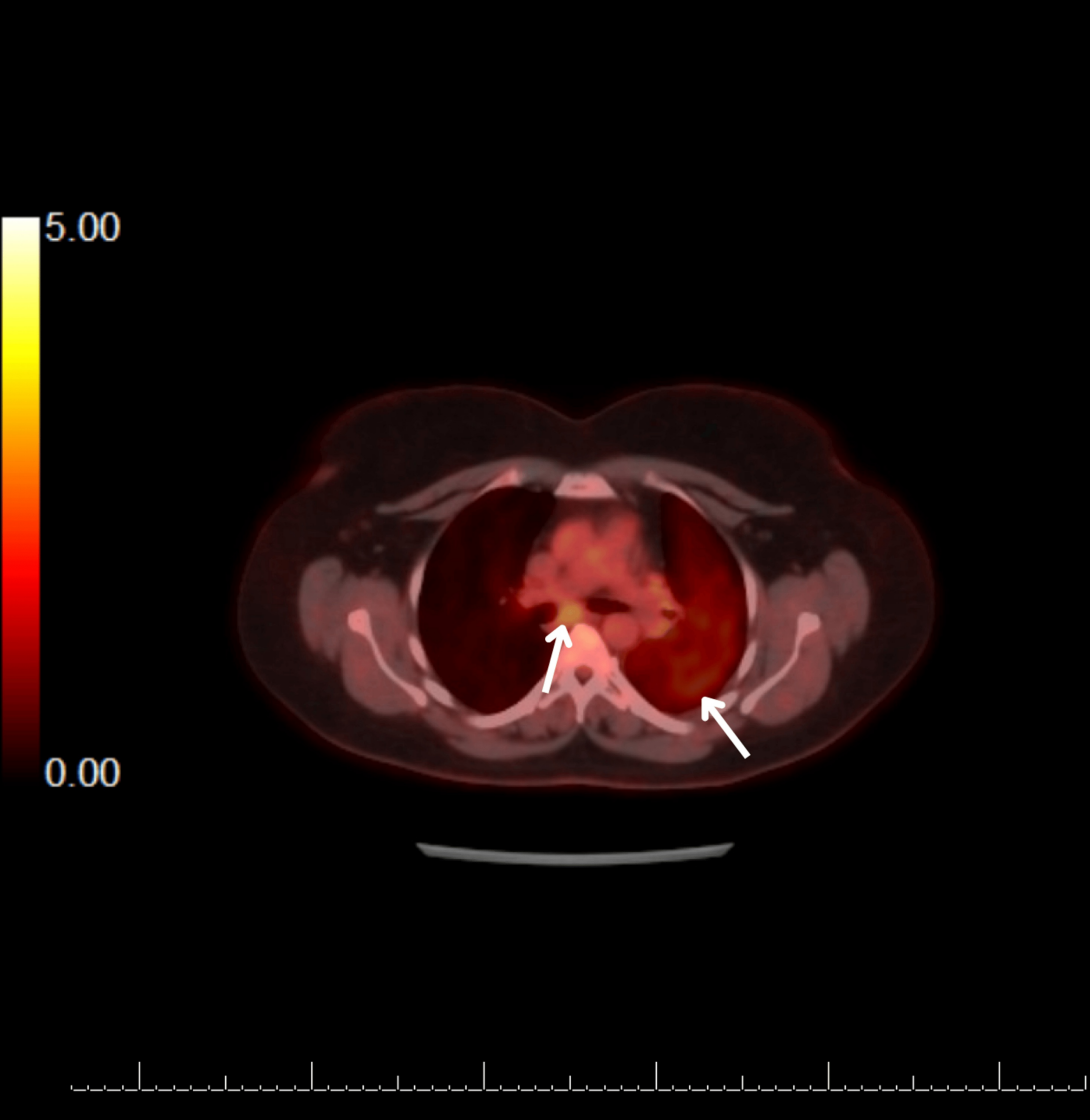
PET-CT image showing FDG-avid uptake in the left lung and mediastinal lymph nodes. FDG: fludeoxyglucose
